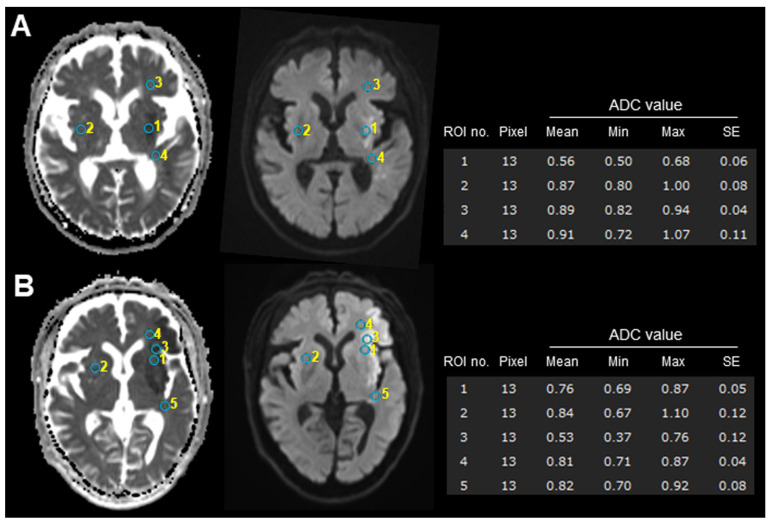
Examples of ROI measurement with (A) and without (B) subIS on admission MRI. Infarction areas reveal high signal intensities on diffusion-weighted MRI (left) and show low signal intensities on ADC map (right). ADC values of circular-shaped ROIs placed on subinsular territory (no. 1) in subIS (A) were lower than those of other case (B). Lower ADC values of infarcted areas (no. 1 in (A) and no. 3 in (B)) were observed, compared to adjacent subcortical white matter or contralateral side. See Supplementary Material for each patient detail. ADC, apparent diffusion coefficient; ROI, region of interest.
